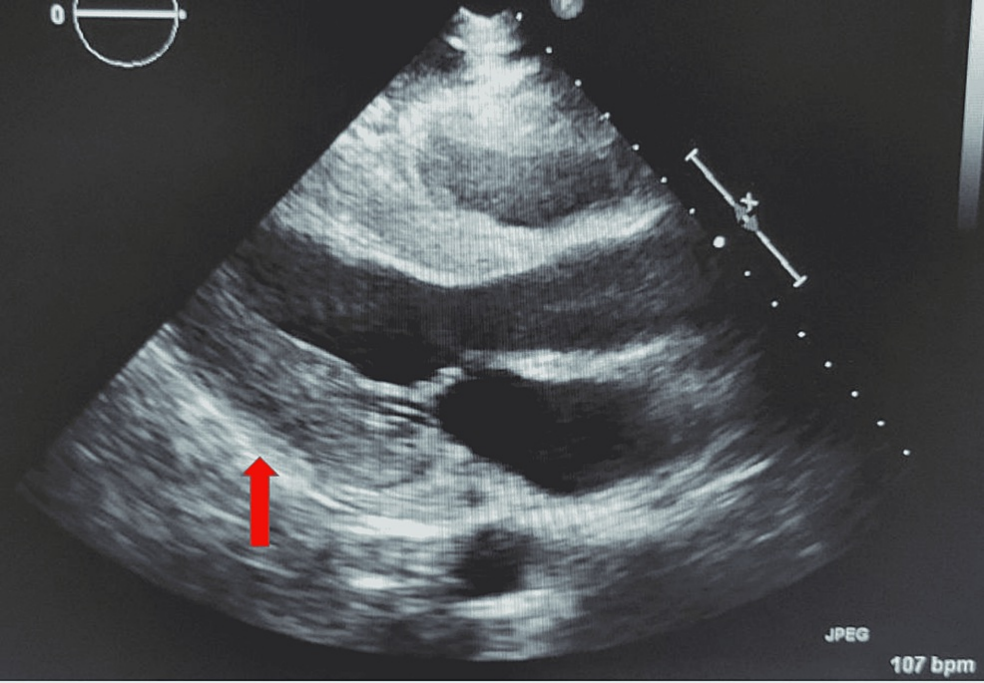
Echocardiogram post pericardiocentesis showed no pericardial effusion (red arrow).
